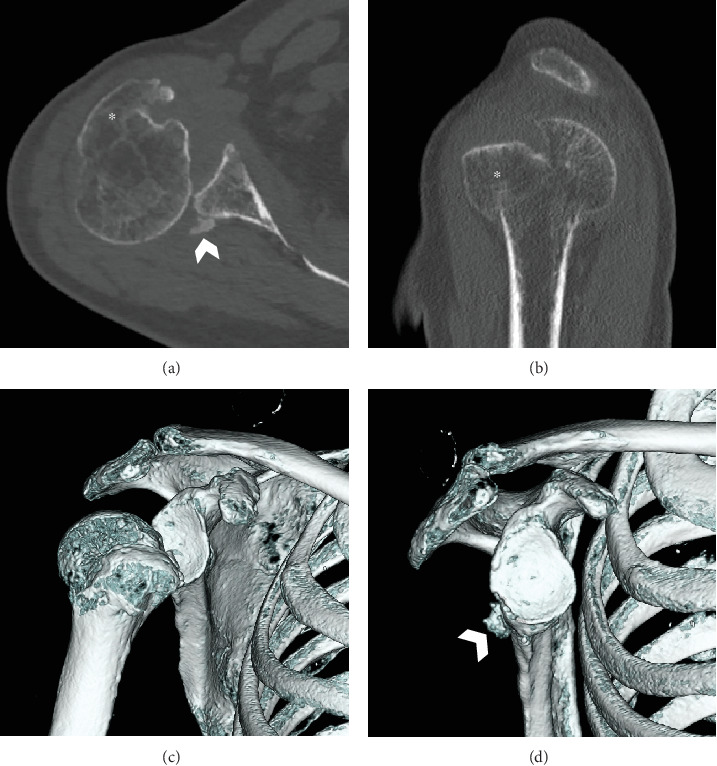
Preoperative CT. (a) Axial and (b) sagittal views showing posterior shoulder dislocation with a malunited greater tuberosity and a small glenoid fracture of the posterior portion. (c, d) 3D reconstruction of the CT images. (d) The humerus was removed to observe the glenoid. Asterisk: greater tuberosity; white arrowhead: glenoid fracture. CT: computed tomography; 3D: three dimensional.
